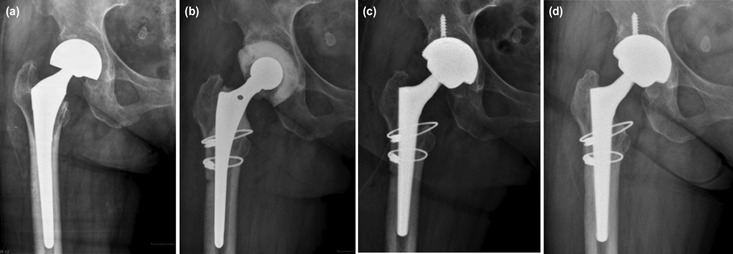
(a) Infected bipolar right hip hemiarthroplasty in a female 59-year-old due to MRSE. (b) Insertion of antibiotic cement spacer, extended trochanteric osteotomy was needed to remove the infected femoral stem. (c) Revision THA was performed after 54 days interval period. (d) Radiograph of final follow-up at 33 months, patient has excellent clinical outcome with no sign of infection.
